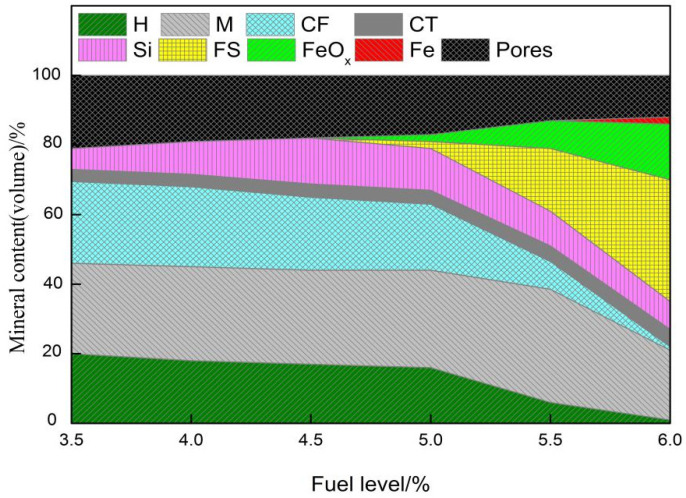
Mineralogy variation of RVT sinter at different fuel levels (H: hematite, M: magnetite, CF: calcium ferrite, Si: silicate (dicalcium silicate, glass, etc), CT: perovskite, FS: fayalite).
